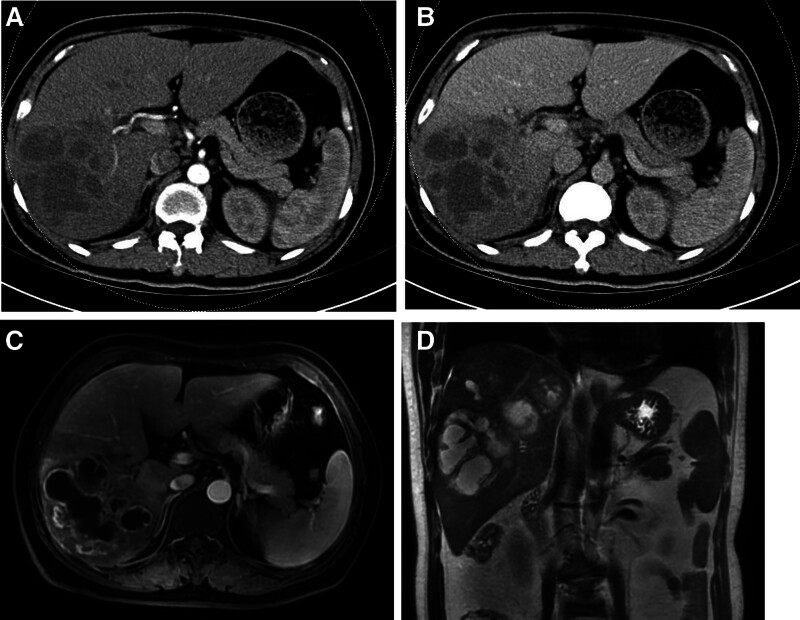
Contrast-enhanced CT (A, B) and MRI (C, D) demonstrating a solid-cystic hepatic mass. CT = computed tomography, MRI = magnetic resonance imaging.
